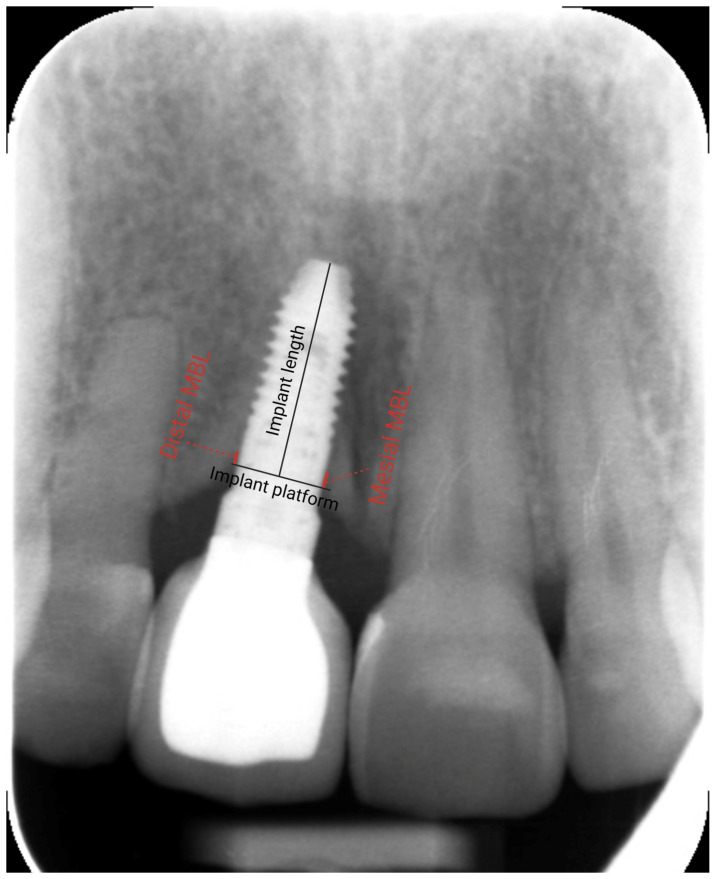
Periapical radiograph showing the measurement of marginal bone level. The known implant length was used to calibrate the radiographic measurements. Then, the mesial and distal marginal bone levels (MBLs) were measured.
